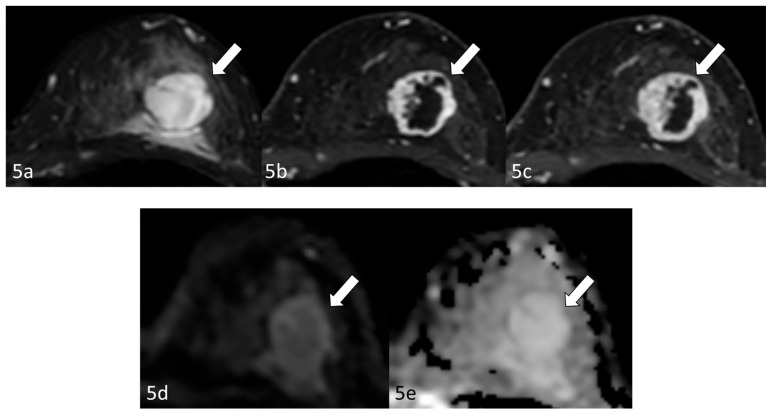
Magnetic resonance imaging (after 11 years): Magnetic resonance imaging (MRI) demonstrated a well-circumscribed round mass (arrow) with high signal intensity on fat-suppressed T2-weighted images (a). Dynamic contrast-enhanced imaging demonstrated spatially heterogeneous enhancement spreading from the periphery to the center, with a fast-plateau enhancement pattern in the peripheral portion and a medium persistent enhancement pattern in the central portion (b,c). The mass (arrows) showed high signal intensity on diffusion-weighted imaging and a high apparent diffusion coefficient value (d,e).
